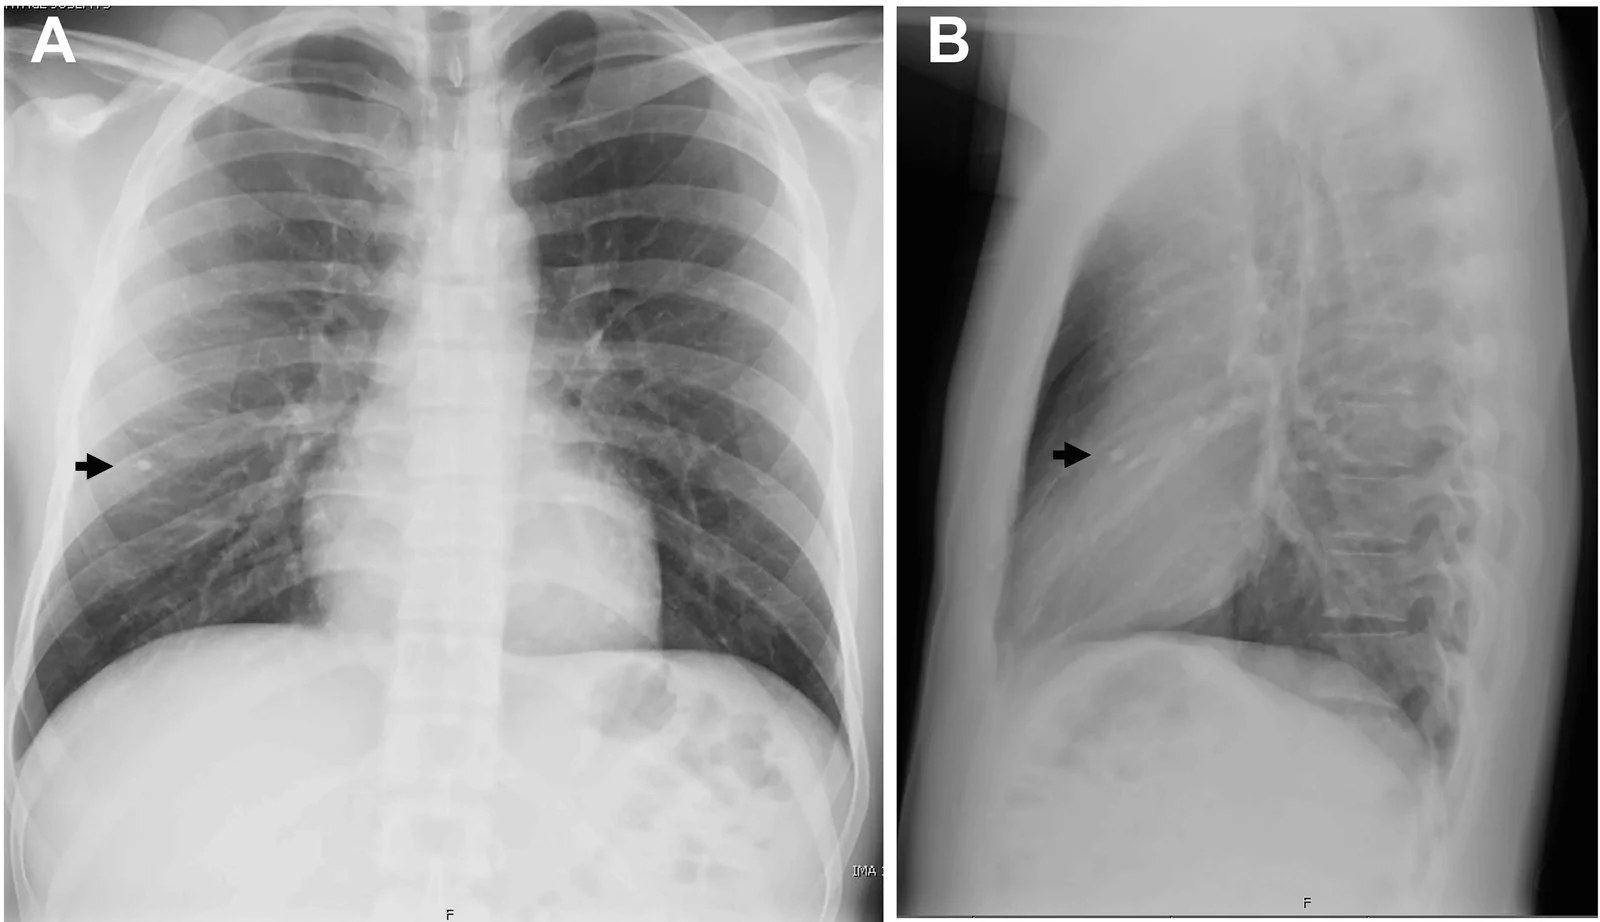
PA and lateral chest X-ray from one year before presentation A single calcified granuloma (arrow) is present in the right middle lobe, and probable hilar lymph node calcifications are seen, without acute abnormality. PA: posterior-anterior
